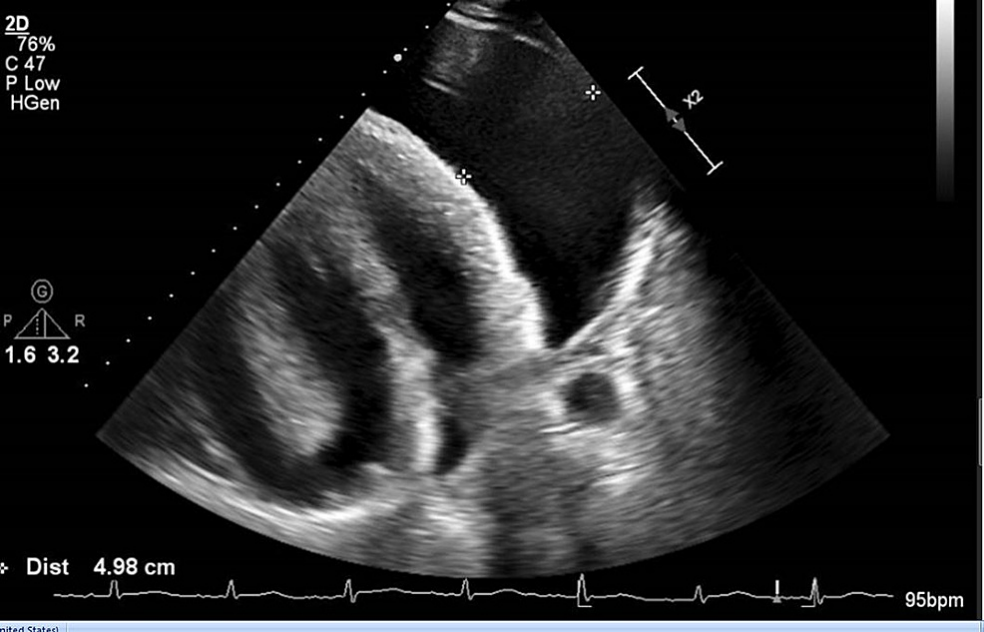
Transthoracic echocardiogram showing large pericardial effusion
